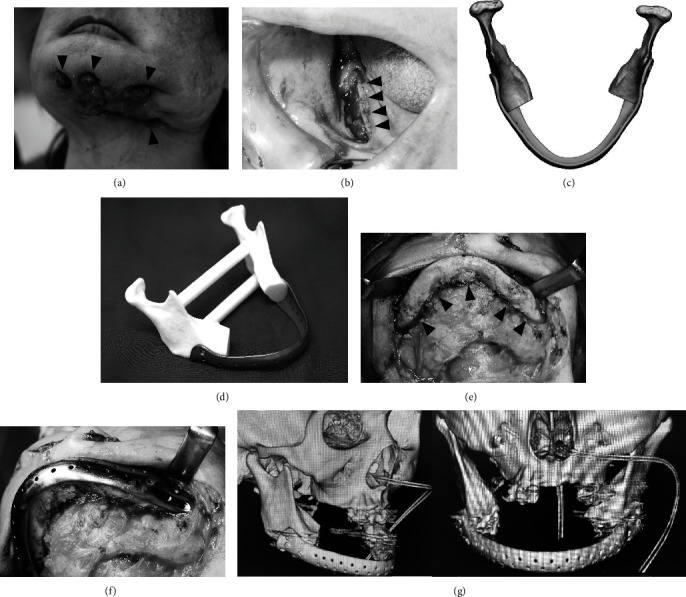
Case 2. (a) Preoperative extraoral photograph. Four large fistulas are observed extending from the chin to the left mandible (arrowhead). (b) Preoperative intraoral photograph. Mandibular bone exposure is observed in the right lower gingiva (arrowhead). (c) Three-dimensional (3D) design of the reconstruction plate. (d) 3D model and reconstruction plate. (e) Intraoperative photograph. The mandible bone is clearly indicated (arrowhead). (f) Confirming the plate on the mandible bone. (g) Postoperative computed tomography. The adaptation between the plate and bone was acceptable.
